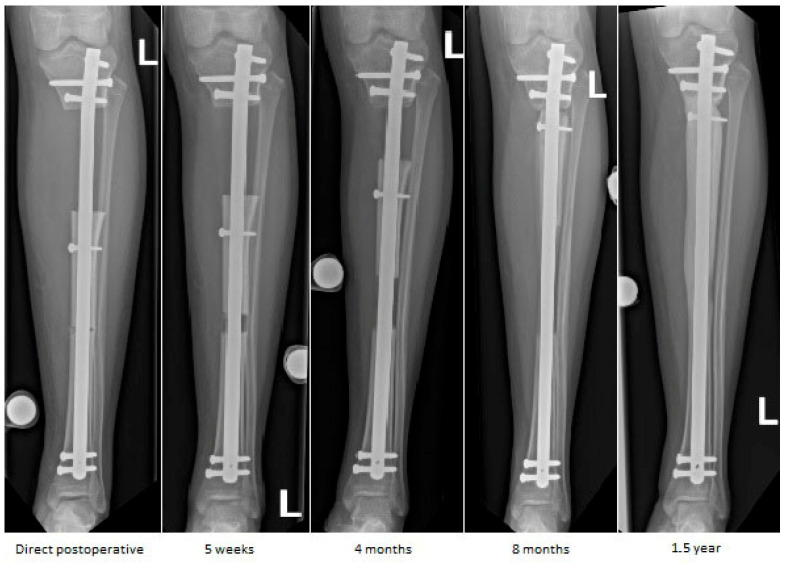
Sequential anteroposterior radiographs following retrograde tibial bone transport at direct postoperative, 5 weeks, 4 months, 8 months, and 1.5 years. The initial segmental defect measured 9 cm. Remaining defect measured 8.5 cm at 5 weeks, 7 cm at 4 months, 1.5 cm at 8 months, and fully consolidated at 1.5 years. Progressive transport and callus formation are evident, with complete restoration of tibial length and alignment at 1.5 years. Distances are approximate due to image scaling.
